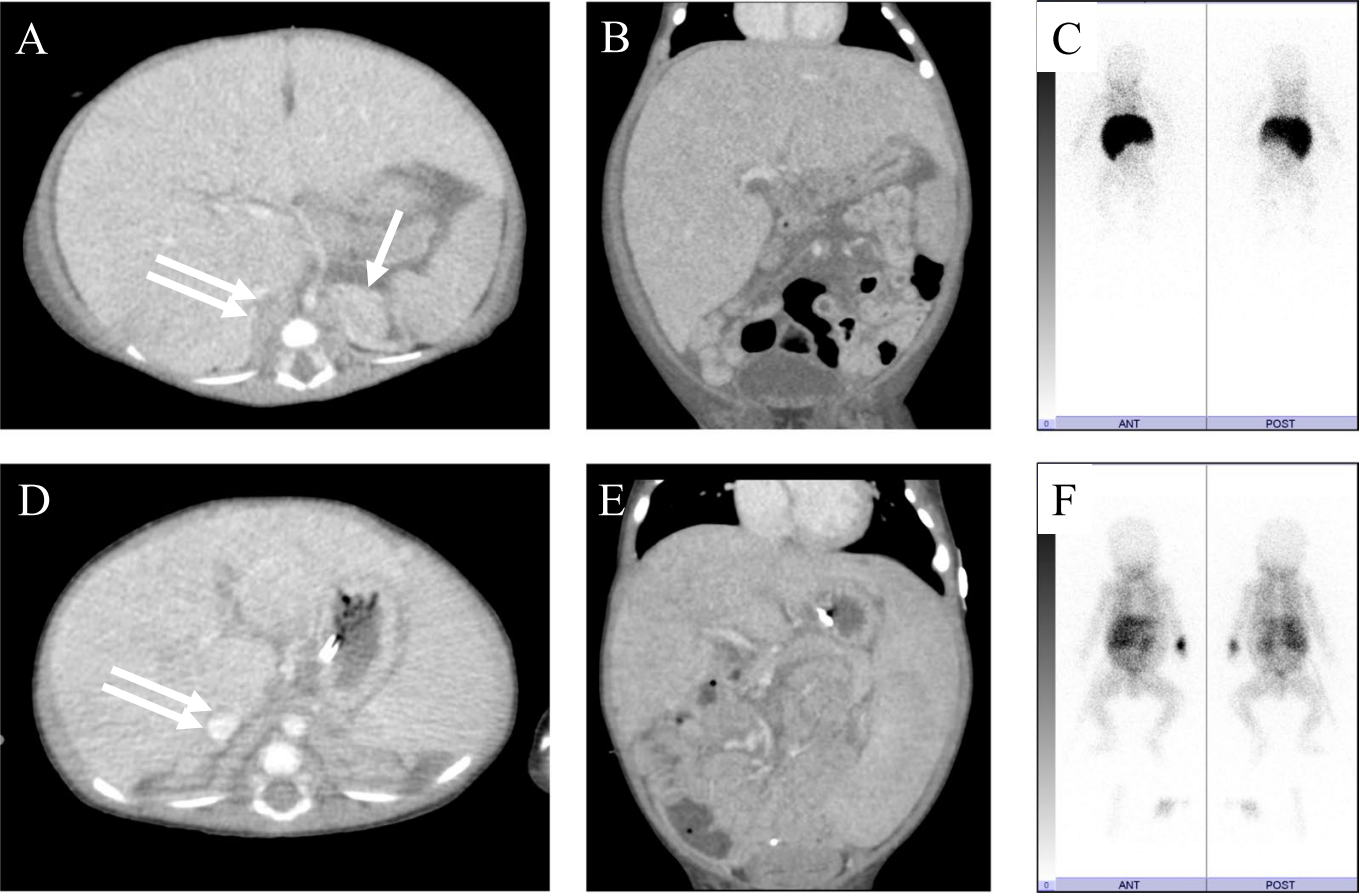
Enhanced computed tomography scan and iodine-123 metaiodobenzylguanidine scintigraphy on admission (A–C) and after chemotherapy (D–F). The single arrow indicates left adrenal gland swelling, and the double arrows indicate the compressed inferior vena cava by the enlarged tumorous liver. Note that the enlarged liver (B) changed to a normal size (E) after chemotherapy. D Left adrenal gland swelling disappeared and the inferior vena cava was relieved by improvement of the hepatomegaly. Iodine-123 metaiodobenzylguanidine scintigraphy on admission (C), significant uptake was seen in the liver, but minimal uptake was seen in the left adrenal gland. F After chemotherapy, the significant uptake in the liver disappeared
